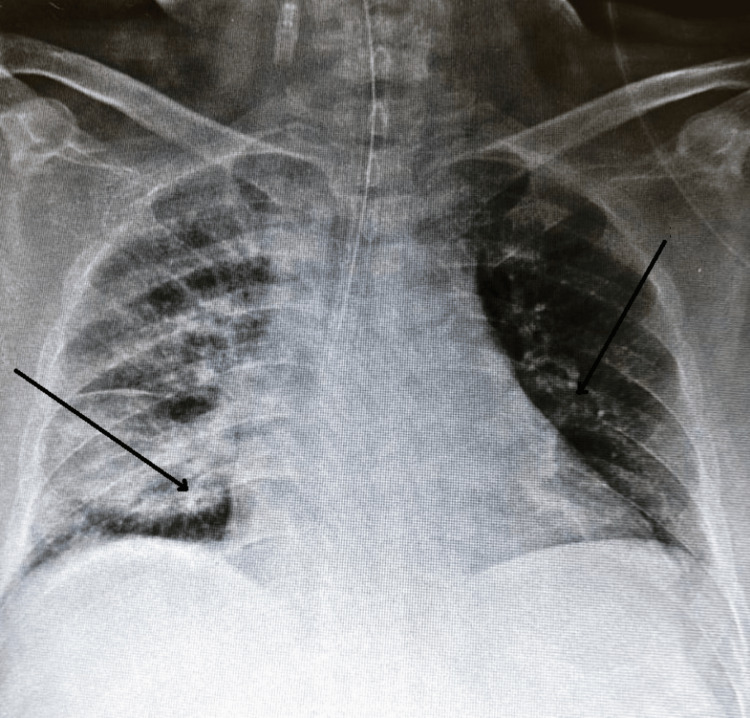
Chest X-ray image with known bronchiectasis
